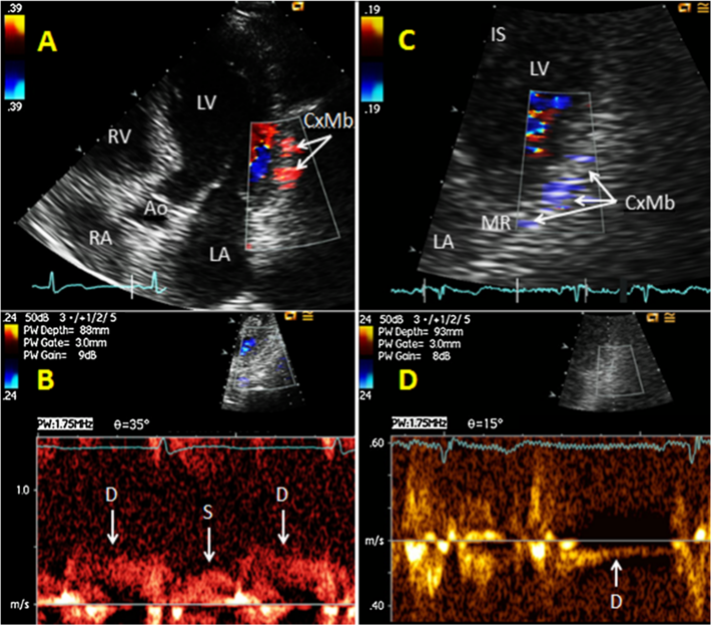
Examples of anterograde and retrograde flow in marginal branches of the left circumflex coronary artery. In modified apical four chamber views, a marginal branch of the left circumflex coronary artery (CxMb) is imaged by colour Doppler mapping with matching spectral Doppler tracings of blood flow: (A,B) The CxMb is seen with anterograde flow. (C,D) The CxMb is seen with retrograde flow. Ao = aortic valve and ascending aorta, D = spectral Doppler tracings of diastolic coronary blood flow, IS = interventricular septum, LA = left atrium, LV = left ventricle, MR = mitral ring, RA = right atrium, RV = right ventricle, S = spectral Doppler tracings of systolic coronary blood flow.
